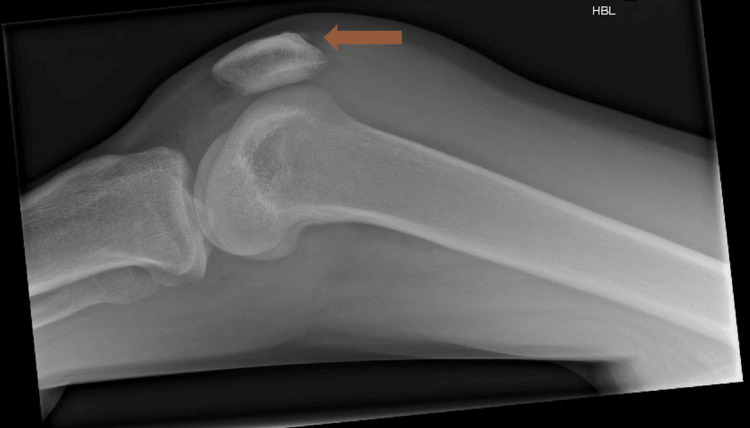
Left knee X-ray (AP, horizontal-beam lateral) demonstrating mild reduction of knee joint spaces suggesting early degenerative changes with small to moderate sized effusions in suprapatellar joint recesses (denoted by orange arrow)
